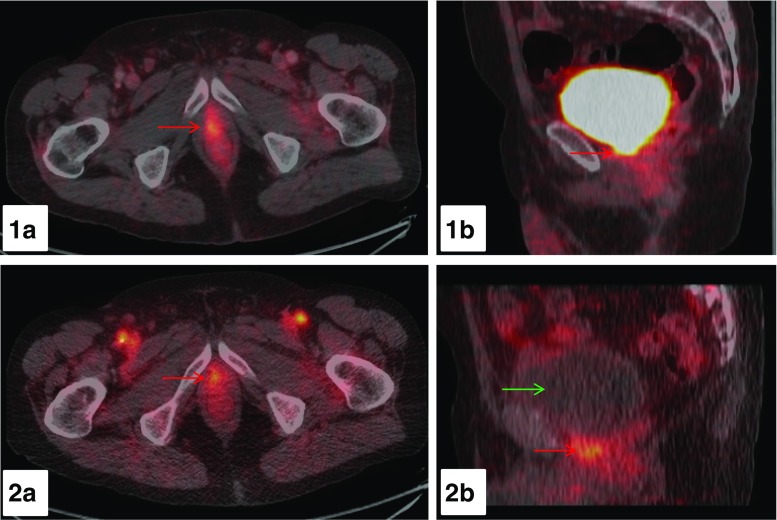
^68^Ga-PSMA-11 PET/CT images of a 72-year-old PC-patient with BR after RP (PSA 4.26 ng/ml). Early dynamic imaging of the pelvis over the first 8 min p.i. and a whole-body scan at 60-min p.i. were performed. At 60-min p.i., a clear distinction between urinary activity within the neck of the urinary bladder and local recurrence is not possible as presented on axial (1a) and sagittal (1b) fused PET/CT images (*red arrow*). In contrast, on the axial and sagittal fused PET/CT-images (2a, 2b) at 4 min p.i. of the early dynamic PET-acquisition a focal tracer accumulation with a SUV_max_ value of 4.45 adjacent to the urinary bladder is visible (*red arrow*) with no tracer uptake in the urinary bladder present (*green arrow*) consistent with local recurrence
